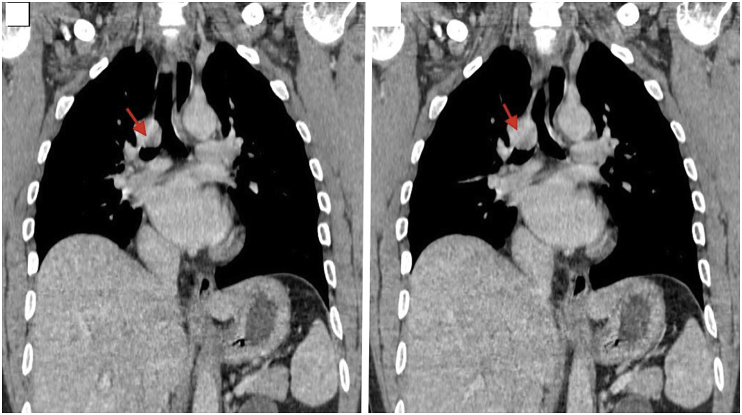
Thoracic CT scan frontal section showing tumor involving the tracheobronchial area.
